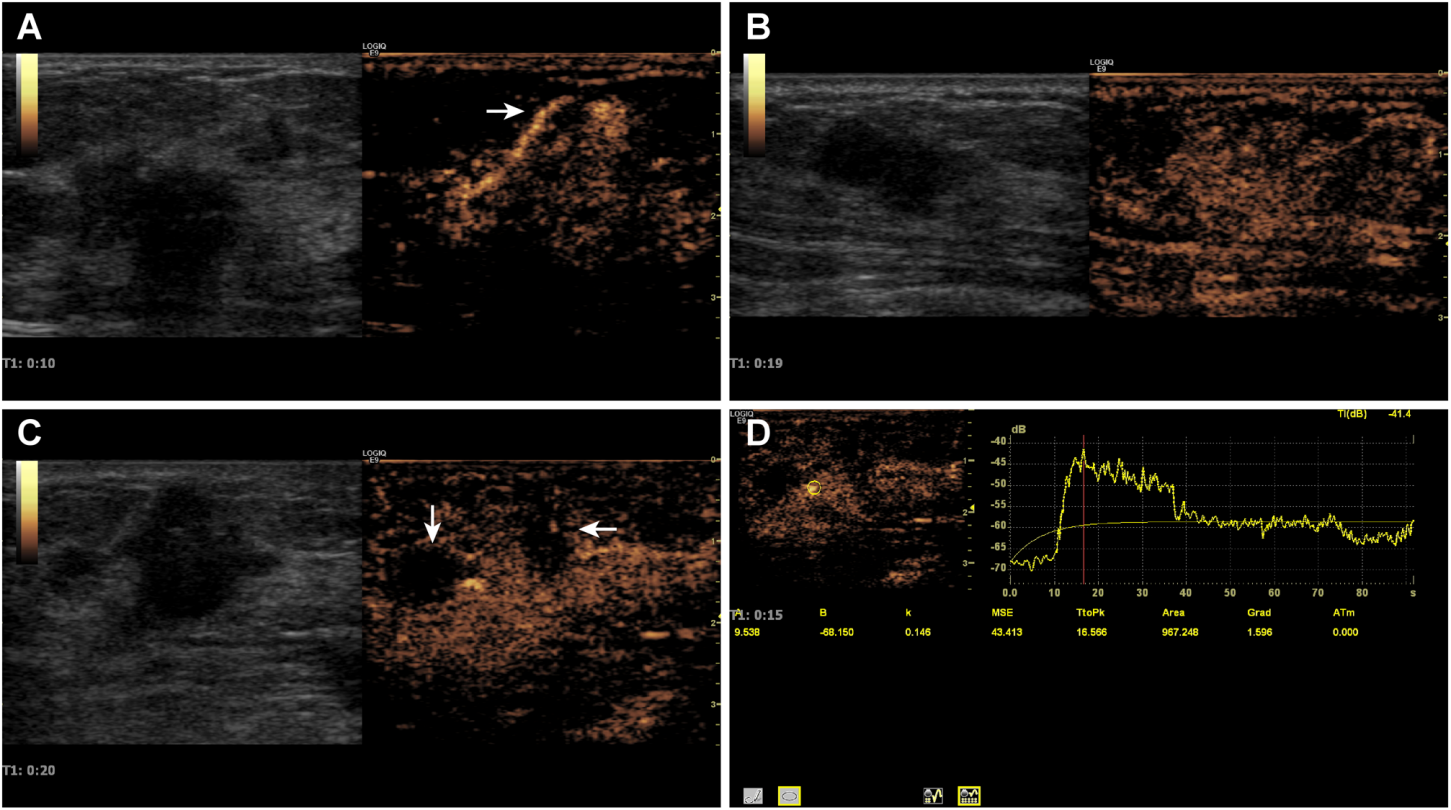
ARFI characteristics of HER-2 (+++) breast cancer. 2a, 2D color Doppler ultrasound image; 2b, VTI image; 2c, VTQ image.
